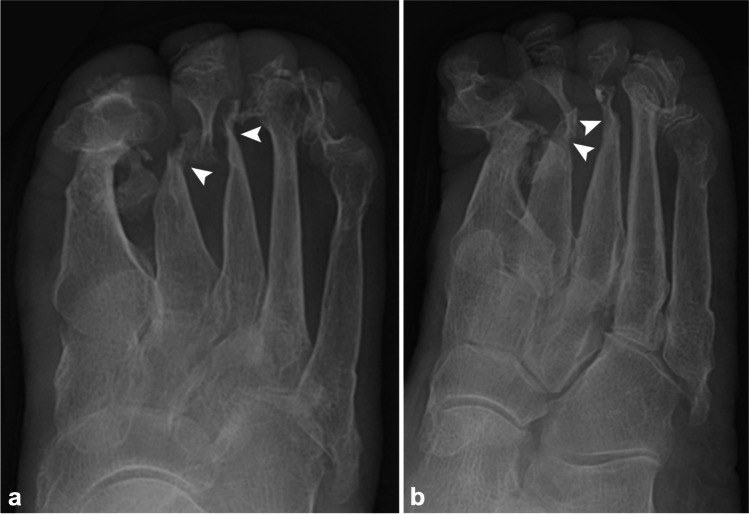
Eighty-five-year-old woman with leprosy (Hansen’s disease) and right foot deformity. AP (a) and oblique (b) radiographs demonstrate arthritis mutilans with osseous destructive changes of the distal metatarsals and phalanges with a “licked candy stick” appearance of the distal second and third metatarsals (*arrowheads*), due to leprosy induced neuropathic arthropathy
